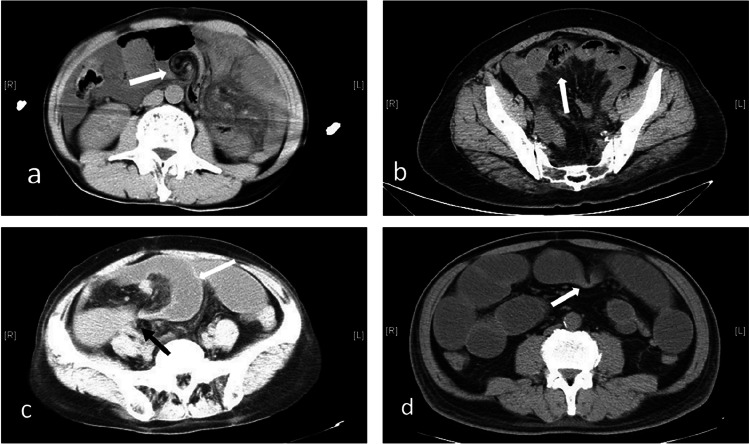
CT signs: a whirl sign, b radial distribution, c U/C-shaped loop (white) and beak sign (black), d fat notch sign
